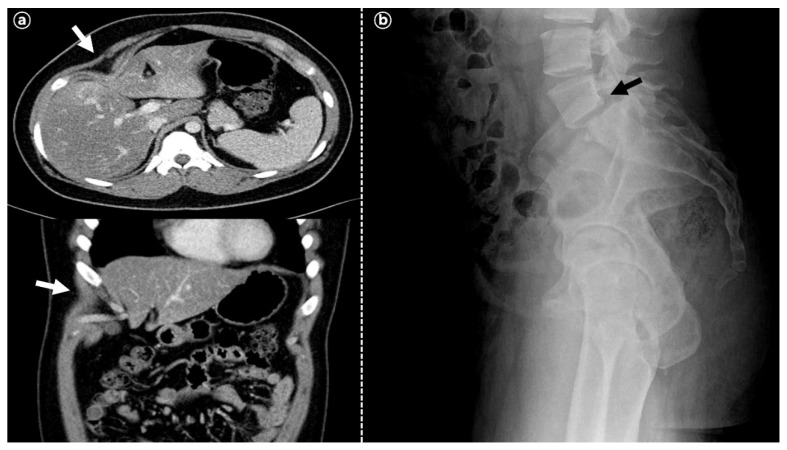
Skeletal deformities of the patient. (a) Axial and coronal CT scan sections showing abnormally curved right 8th rib and costal that directly compress the liver (white arrow); (b) sagittal lumbosacral X ray showing spondylolisthesis of L5-S1 (black arrow).
